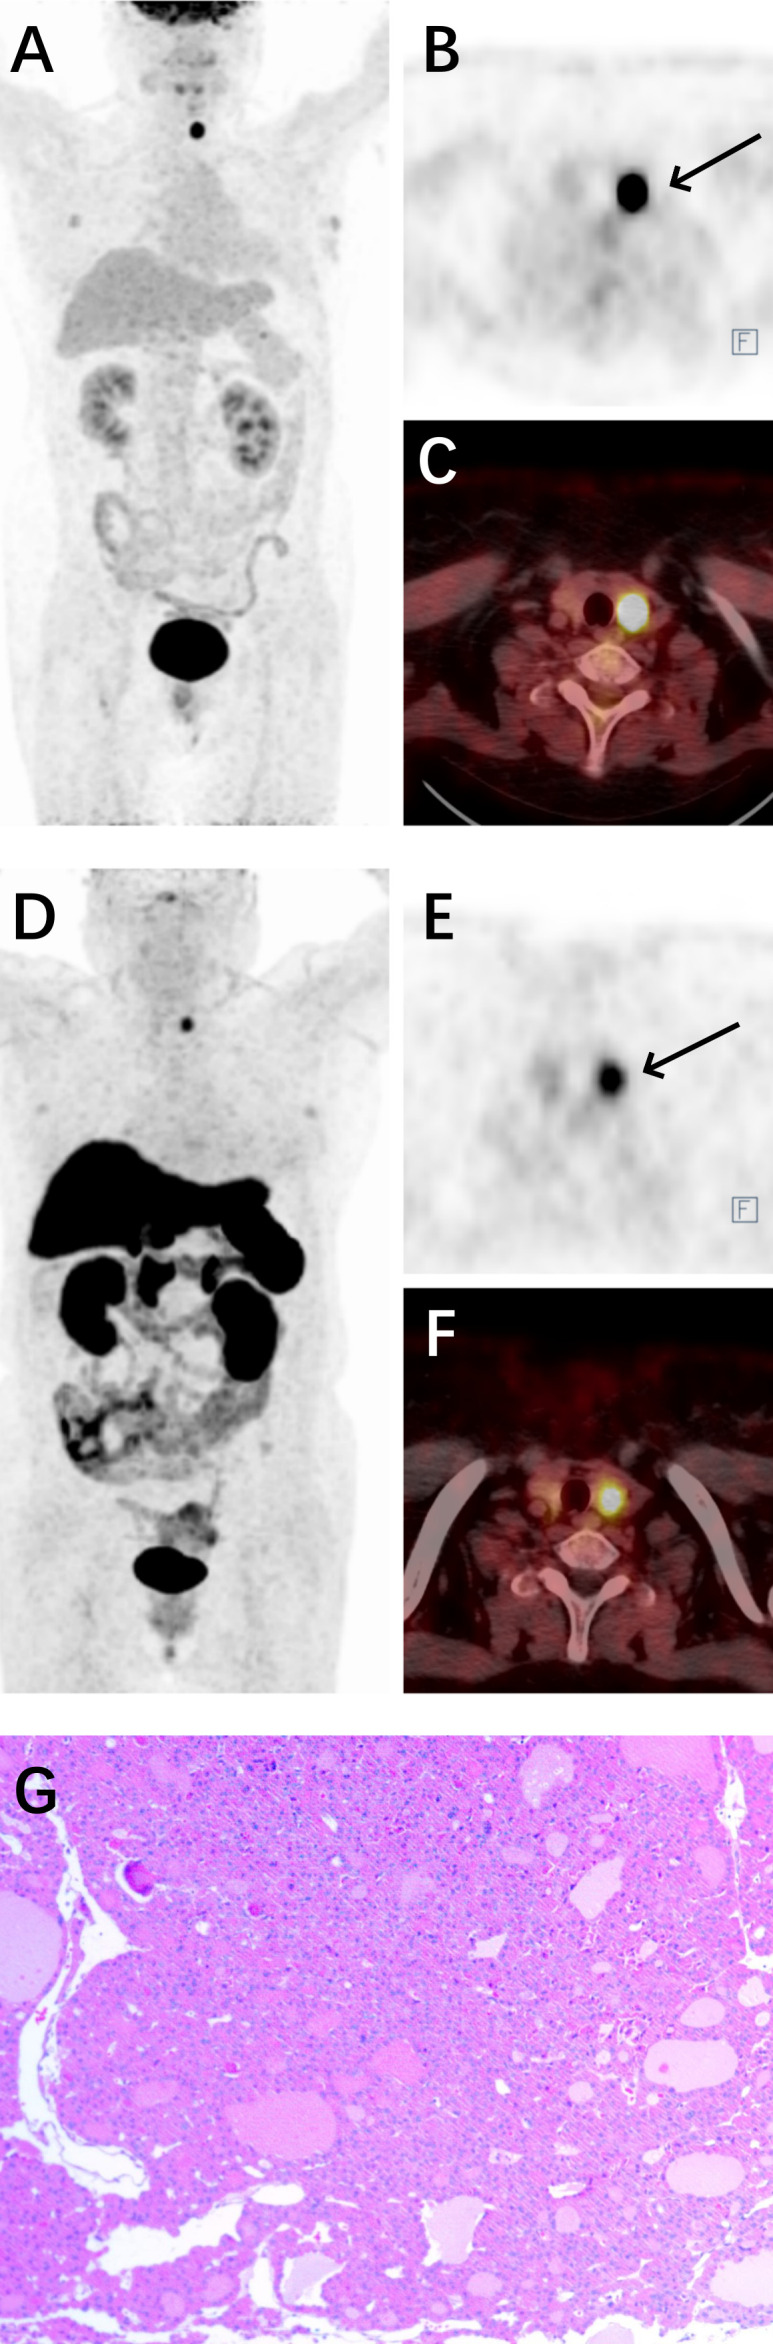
^18^F-FDG combined with ^18^F-OC PET/CT of OA and surgical pathology. (A) Maximum-intensity projection (MIP) image of the patient in ^18^F-FDG PET. (B) Axial view of the tumor in ^18^F-FDG PET (black arrow). (C) Fusion image in ^18^F-FDG PET/CT. (D) MIP image of the patient in ^18^F-OC PET. (E) Axial view of the tumor in ^18^F-OC PET (black arrow). (F) Fusion image in ^18^F-OC PET/CT. (G) Surgical pathology of OA.
